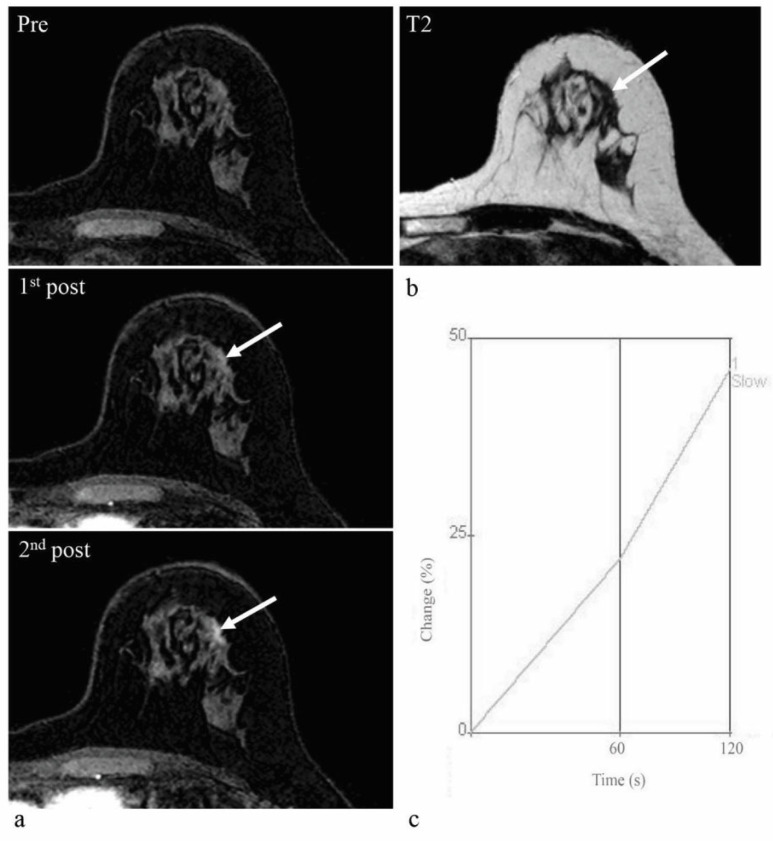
Screening abbreviated breast MRI (AB-MRI) images of a 48-year-old woman with a history of right total mastectomy and left breast-conserving surgery due to bilateral breast cancer. Two years after surgery, pre-contrast and two post-contrast-enhanced axial images (a) show a 0.9 cm irregular enhancing mass (arrows) in the left breast at the 1 o’clock direction. The T2 signal intensity is not high (b). This lesion was assessed as BI-RADS 4B category in the original report. According to the interpretation guideline, it is classified as a suspicious lesion for which biopsy is recommended. However, kinetic analysis shows a low enhancement degree of 22% on the first post-contrast-enhanced image (c). This lesion was detected by second-look ultrasound (US) and confirmed as sclerosing adenosis by US-guided core biopsy. In conclusion, considering kinetic information could have avoided an unnecessary biopsy of this false-positive lesion.
